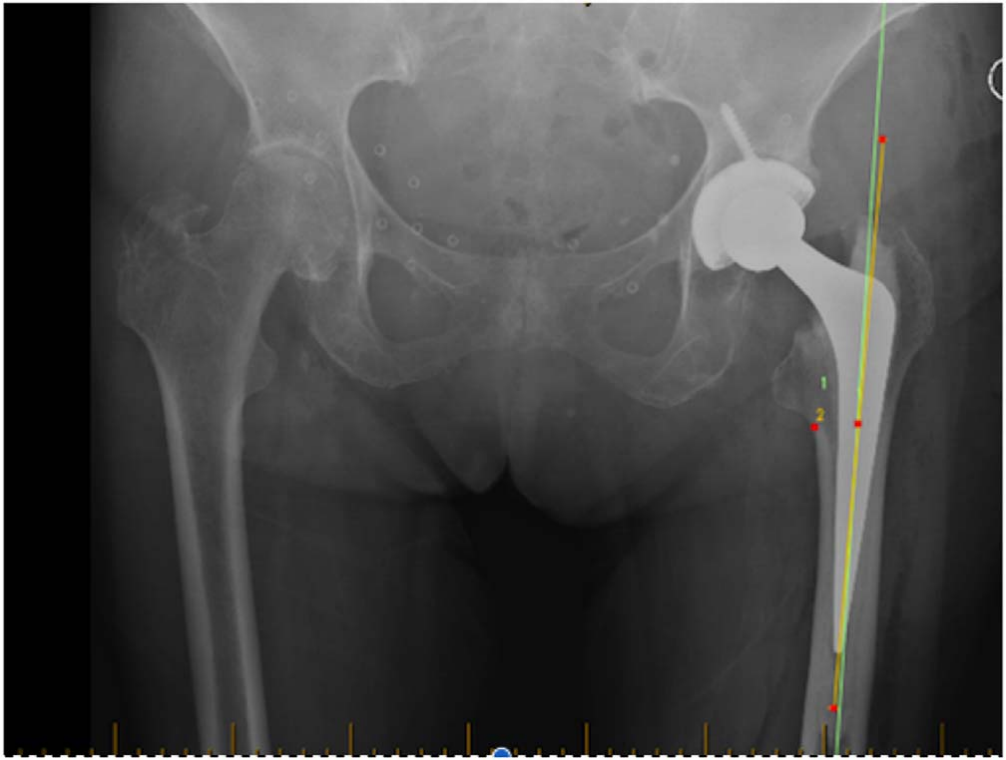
Anteroposterior view of X-ray hip showing a Barrack grade A cementation following bikini total hip replacement
